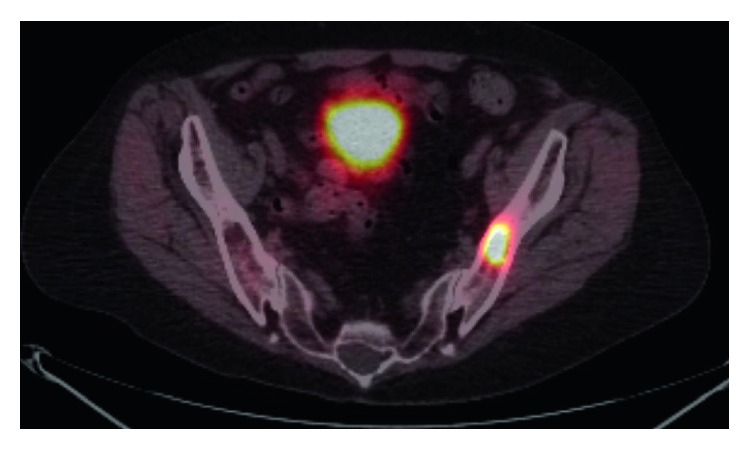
PET CT with hypermetabolic mass within the left iliac bone.
